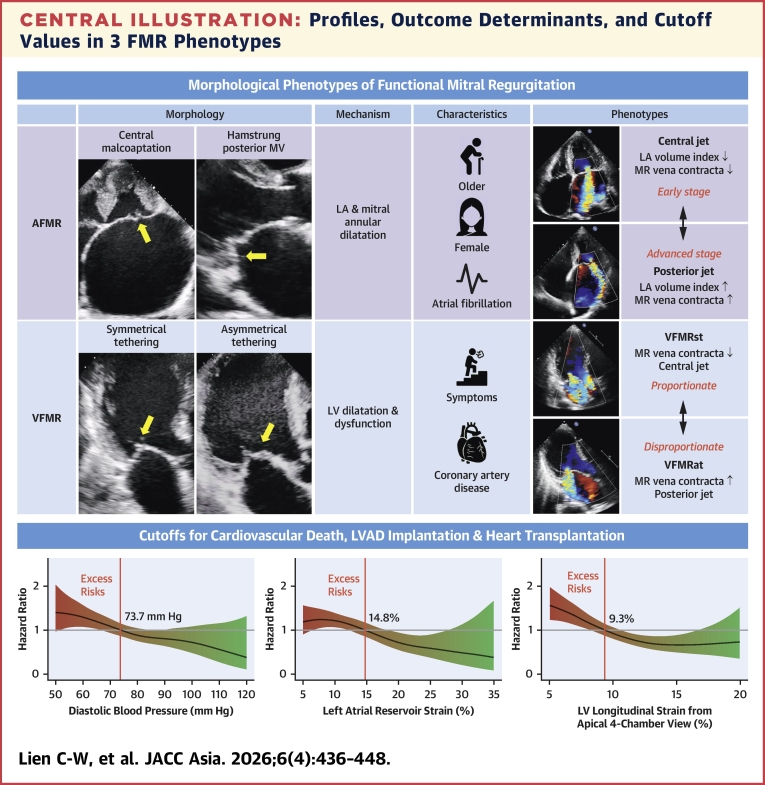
Profiles, Outcome Determinants, and Cutoff Values in 3 FMR Phenotypes Patients with atrial functional mitral regurgitation (AFMR) were older, predominantly female, and with higher prevalence of atrial fibrillation. Two types of AFMR were mitral valve (MV) central malcoaptation with central jet and hamstrung posterior MV with posterior jet. The latter displayed larger left atrial (LA) volume index and mitral regurgitation (MR) vena contracta, suggesting a more advanced stage. Two types of ventricular functional mitral regurgitation (VFMR) were ventricular functional mitral regurgitation with symmetrical tethering (VFMRat) and ventricular functional mitral regurgitation with symmetrical tethering (VFMRst). The latter had larger MR vena contracta, mimicking disproportionate MR. Adjusted spline curves indicated increased risk of the primary endpoint when diastolic blood pressure ≤73.7 mm Hg, left atrial reservoir strain (LASr) ≤14.8%, and left ventricular (LV) longitudinal strain from the apical 4-chamber view ≤9.3%. Yellow arrows indicate the site of malcoaptation or tethering. LVAD = left ventricular assist device.
